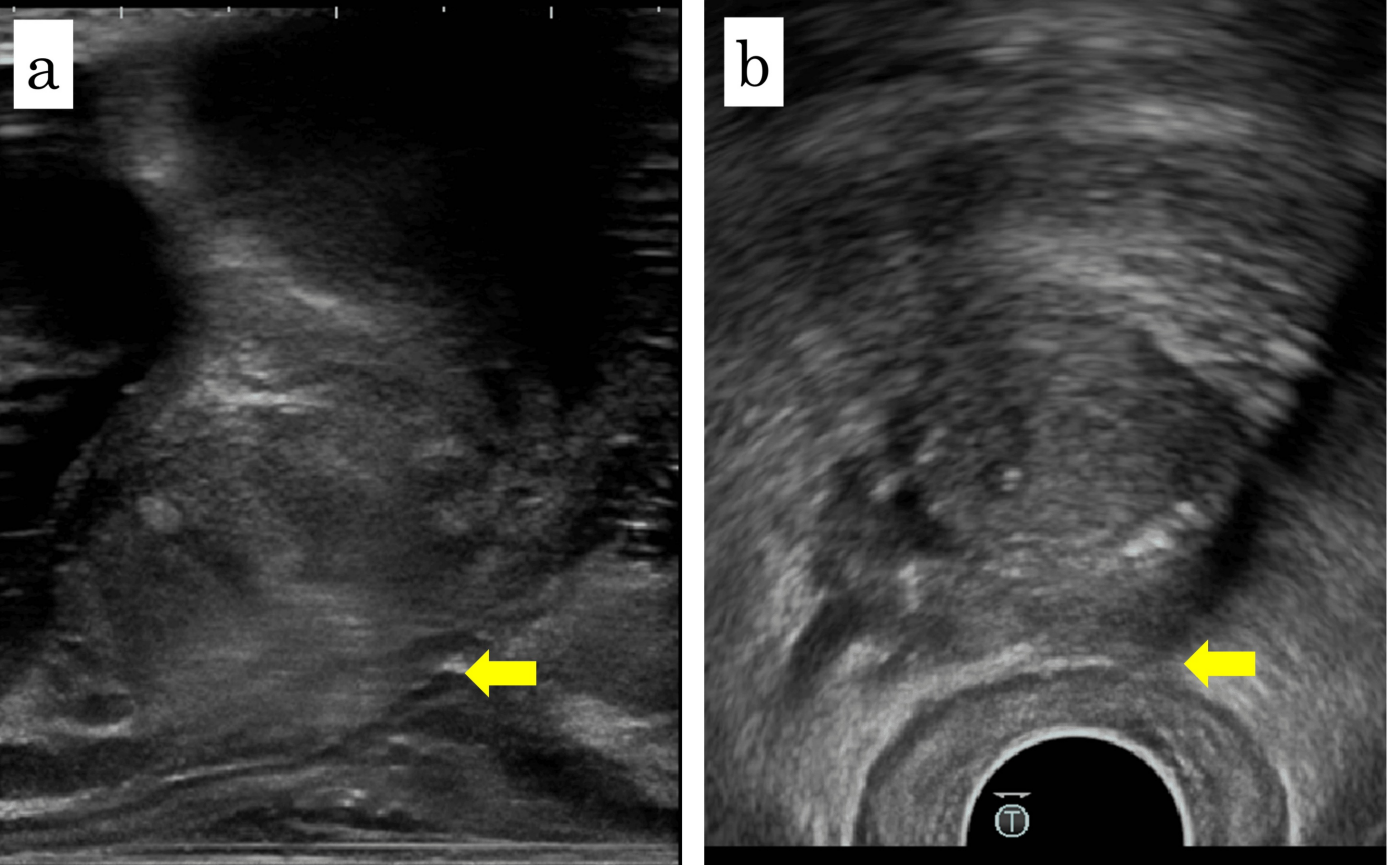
Sagittal and axial TRUS images before spacer placement Sagittal (a) and axial (b) transrectal ultrasound (TRUS) images obtained immediately before spacer placement. Both views show a thin, well-defined Denonvilliers’ fascia (yellow arrows) without evidence of fibrosis or adhesion between the posterior prostate and anterior rectal wall.
